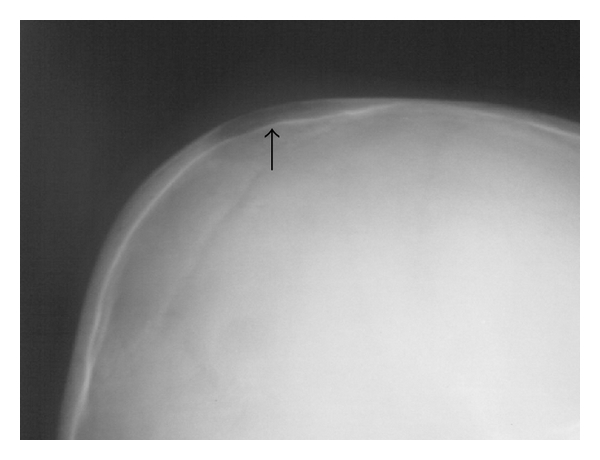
Plain radiograph of the skull taken at time of presentation showing a lytic lesion on the left frontoparietal bone.
